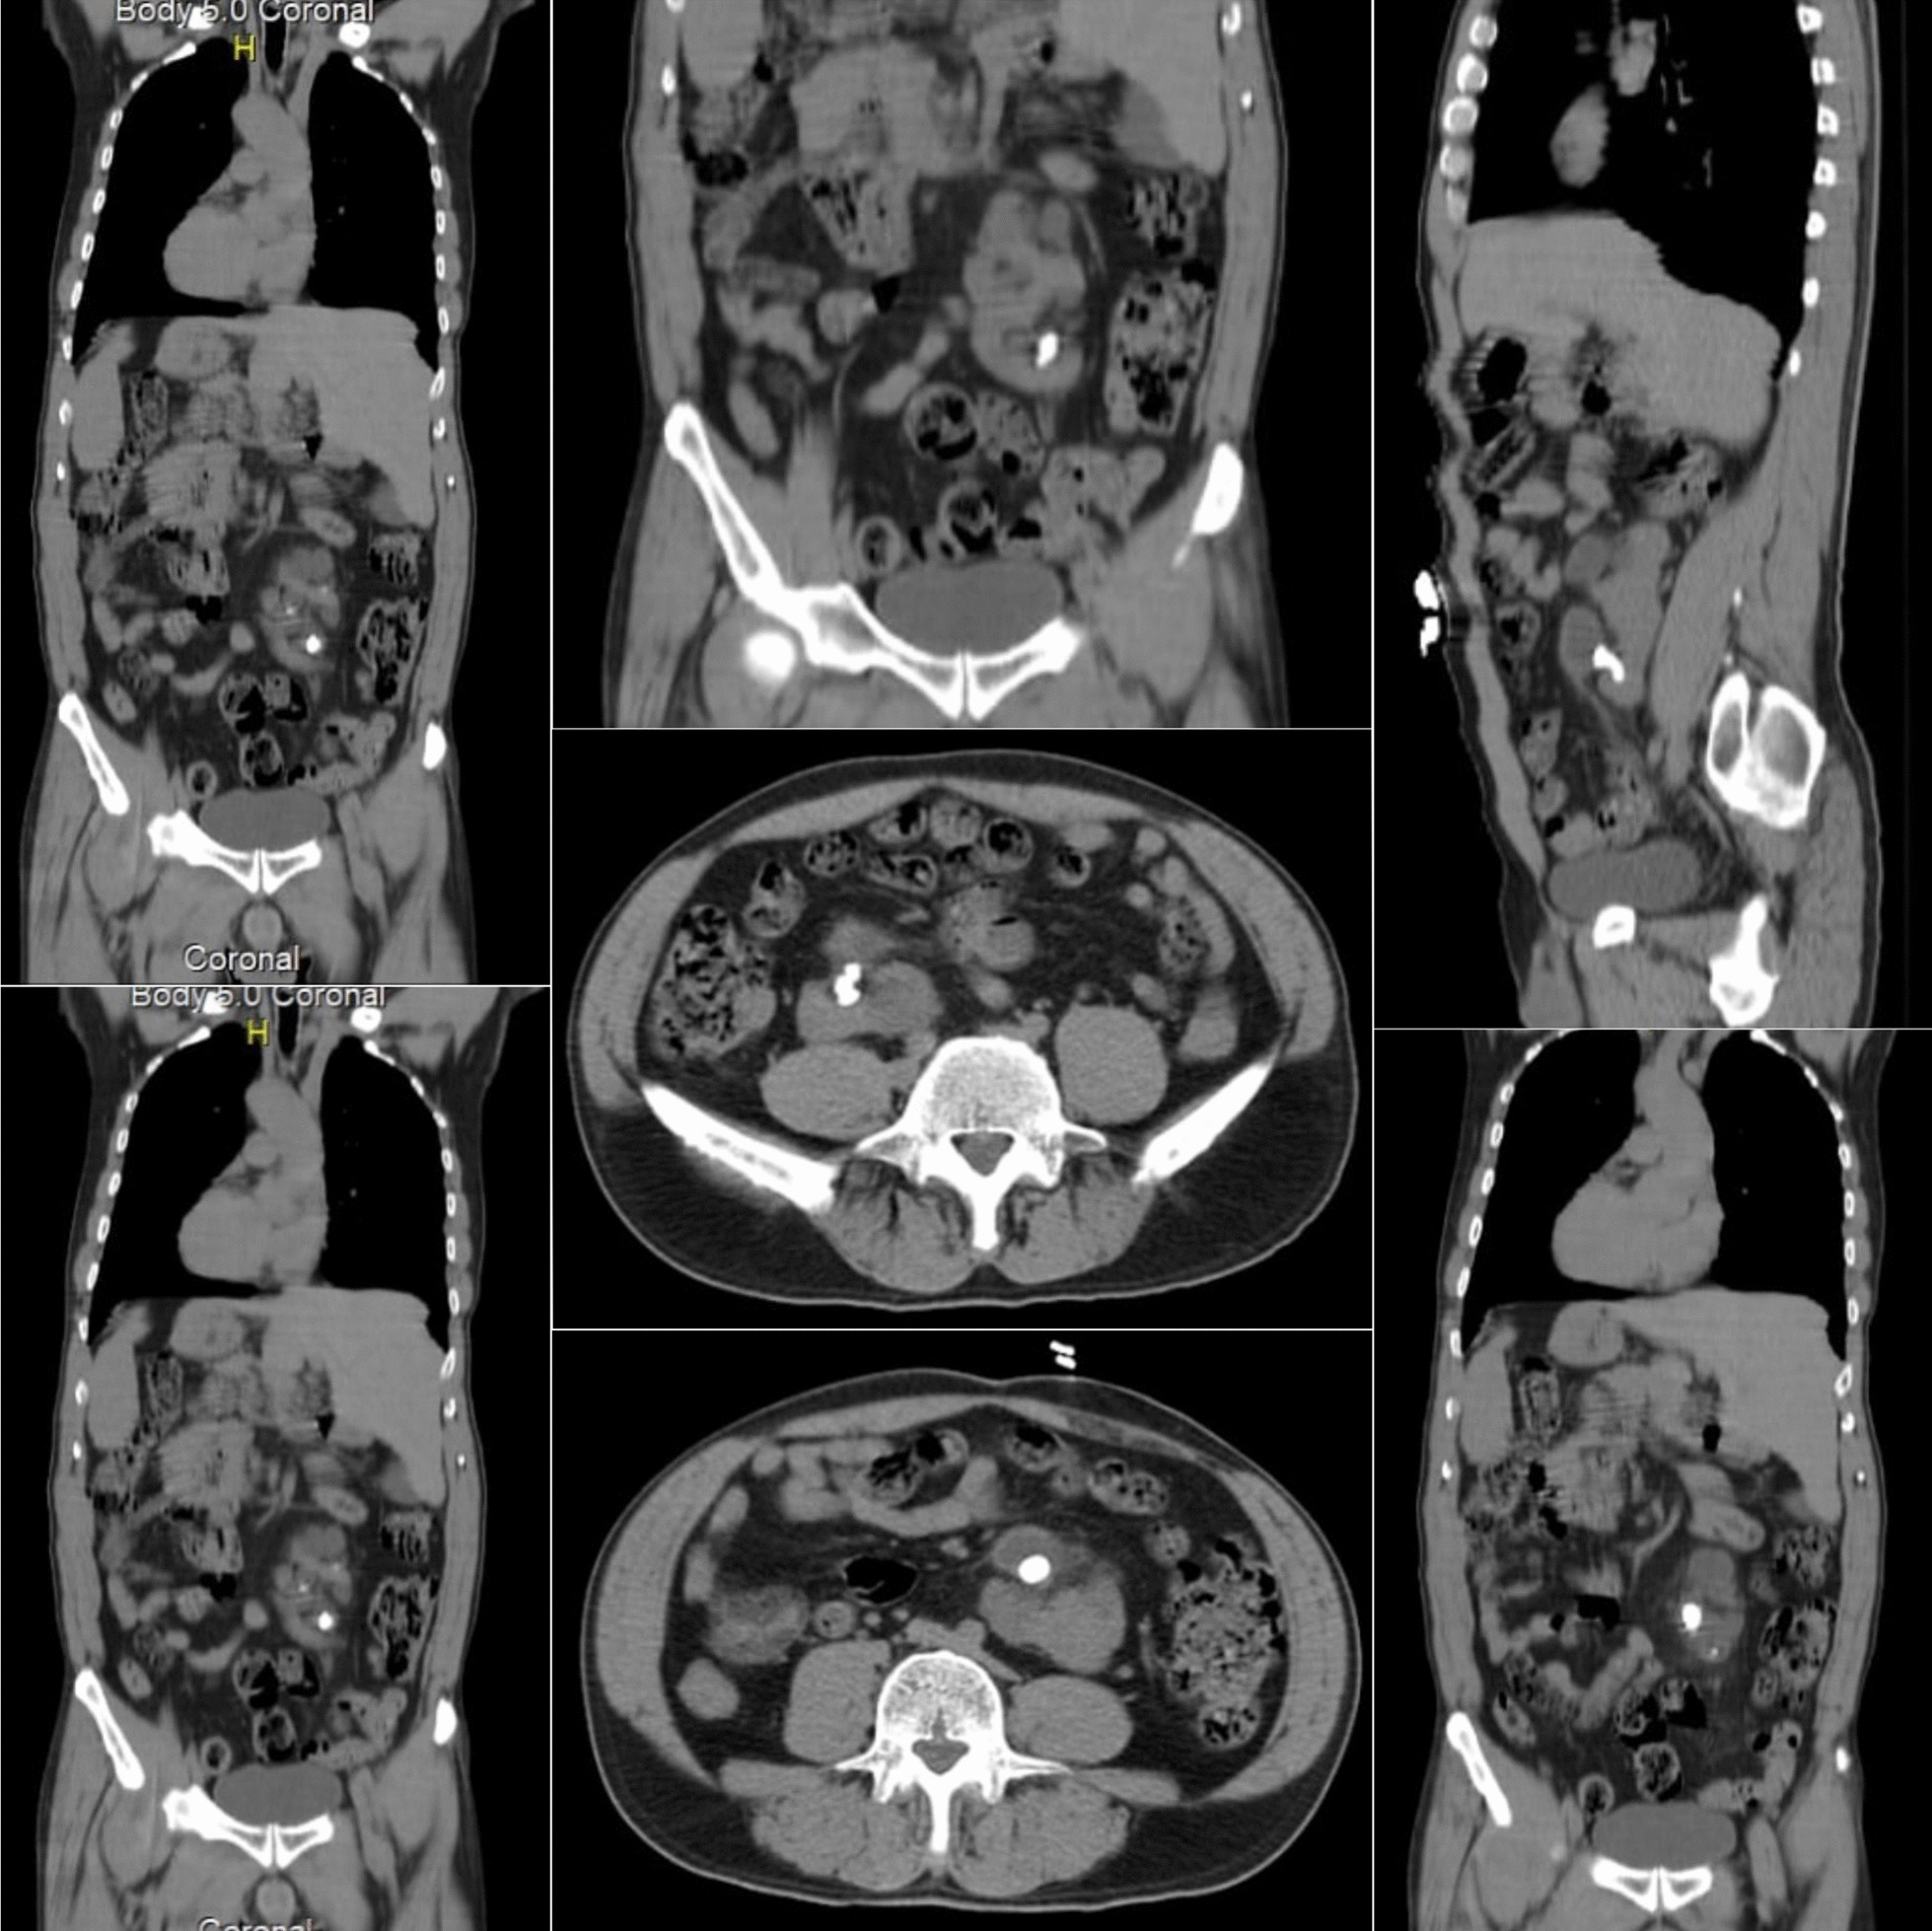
Different planes of CT KUB showing different kidney stones
